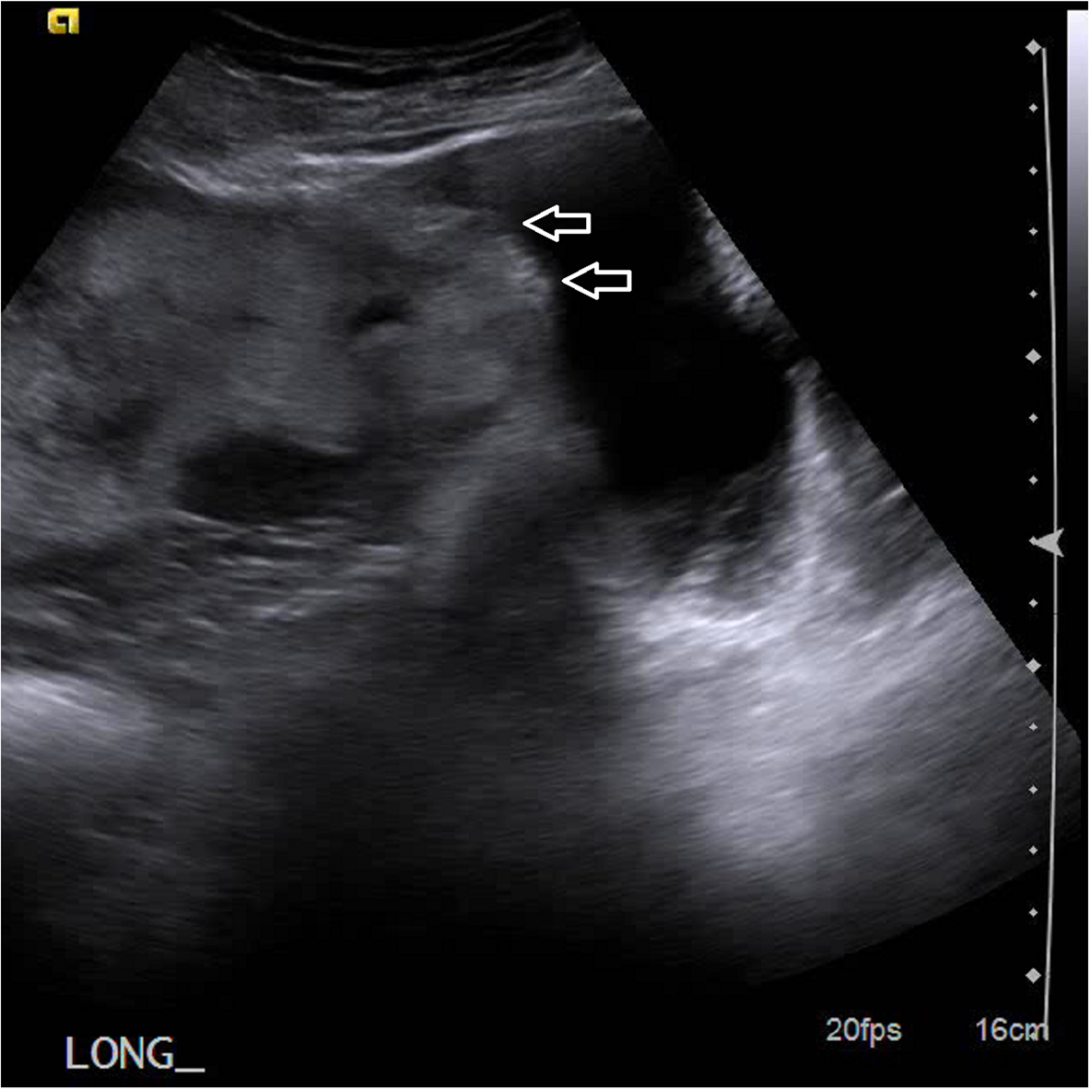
Grey scale sonographic image showing many of the features of PAS, including loss of the retroplacental hypoechoic zone, retroplacental myometrial thickness < 1 mm, and a lower uterine segment echogenic “bulge” (arrows)
